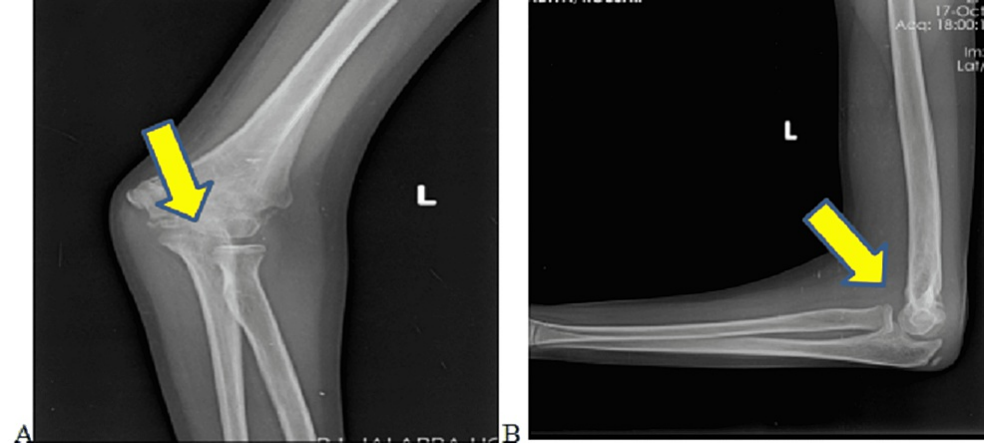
Plain radiographs of the left elbow (A) antero-posterior and (B) lateral views at three months follow-up
